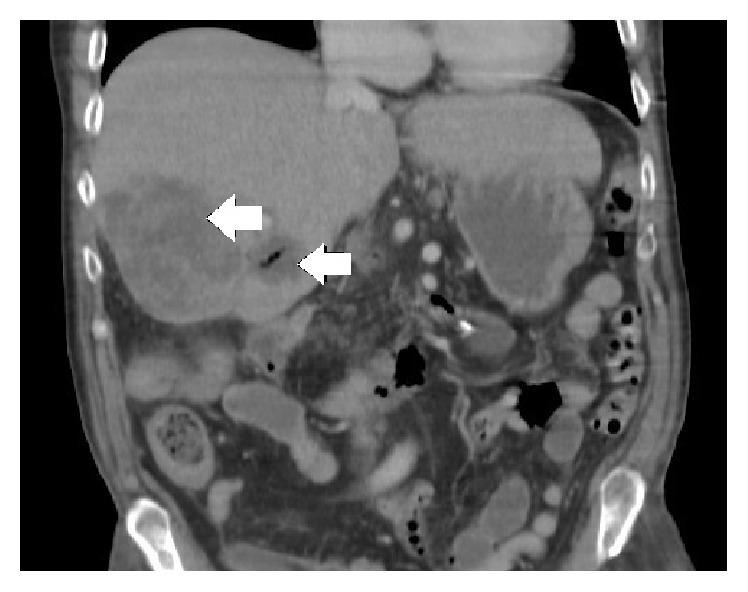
CT showing liver abscess formation within the liver.
